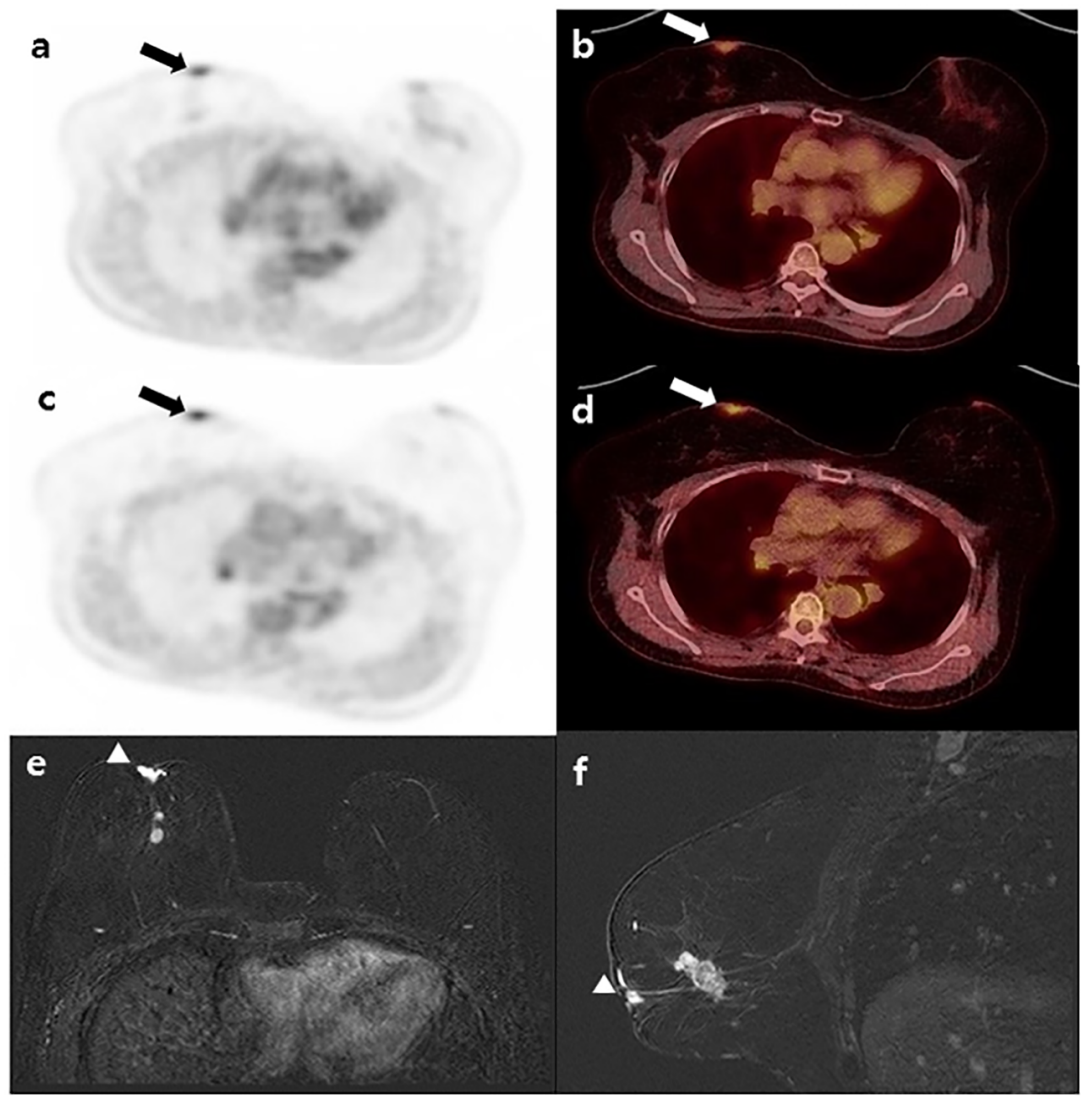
A 51-year-old patient with invasive ductal carcinoma (IDC) in the right breast: (a, b) Early phase ^18^F-FDG PET and fusion PET/CT; (c, d) Delayed phase PET and fusion PET/CT depict focal FDG uptake in the NAC of the right breast (arrow; ROI of NAC showing green color), NAC-SUVR_early_ = 2.35; NAC-SUVR_delay_ = 2.95; (e) Contrast-enhanced MRI with axial view and (f) MRI with sagittal view; MRI shows unilateral NAC enhancement and asymmetric thickening of the NAC (arrowhead). This patient underwent mastectomy, and NAC involvement was pathologically confirmed.
